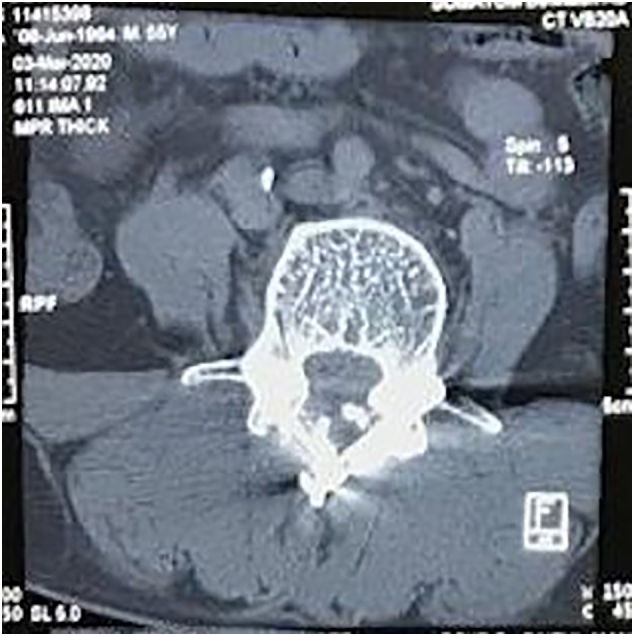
Computed tomography scan of a translaminar screw fixation eight months after surgery. The screws are ideally placed, crossing the facet joint perpendicularly and ending in the base of the transverse process.
